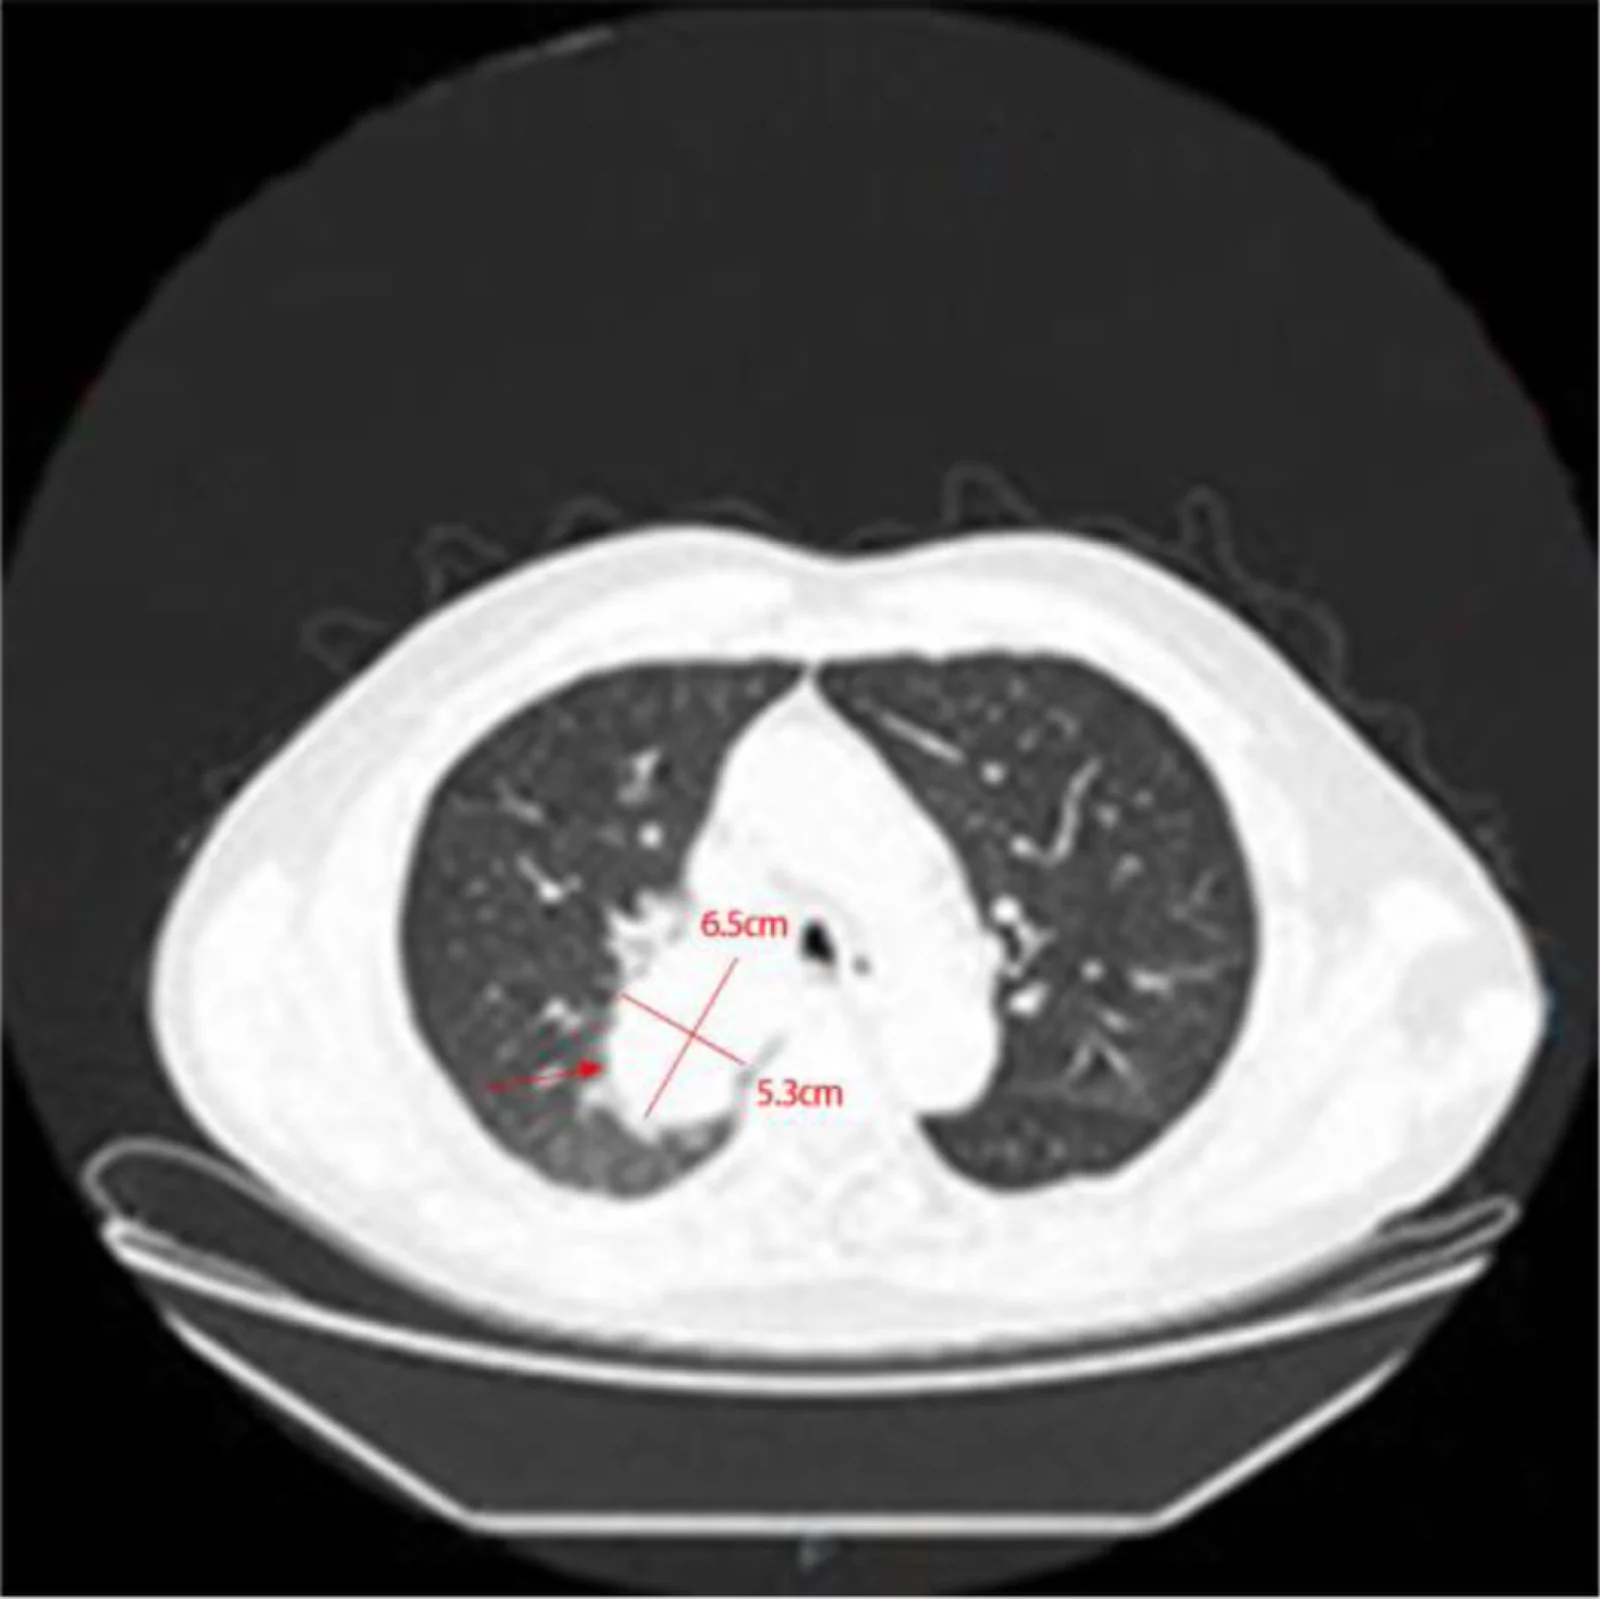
March 2020, lung window and mediastinal window of CT scan showed a 6.5 × 5.3cm right pleural nodule. CT = computed tomography.
